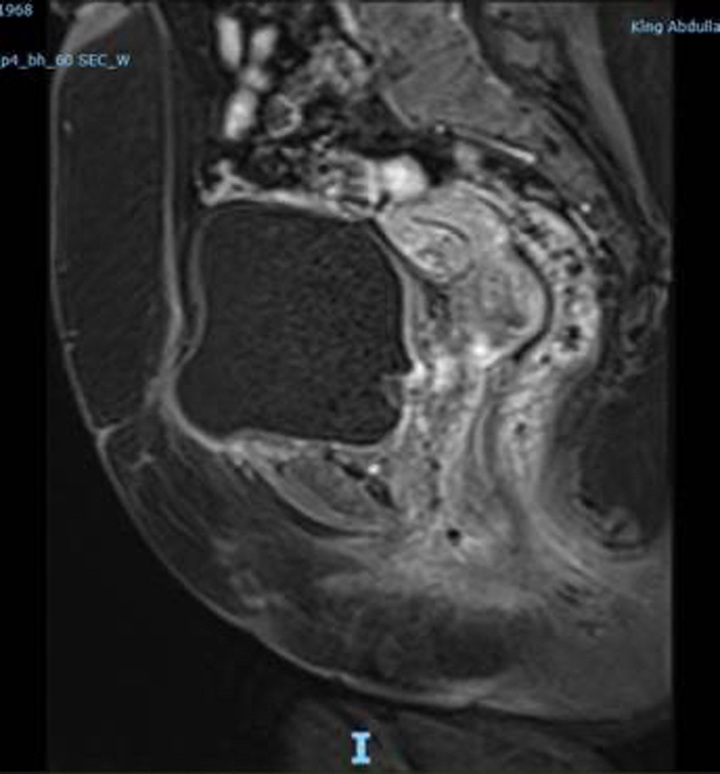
An enhanced pelvic MRI status post removal of the mesh showed small residual fibrosis and granulation tissue in that region with tethering of the posterior bladder wall. No definite leak or signs of fistula.
